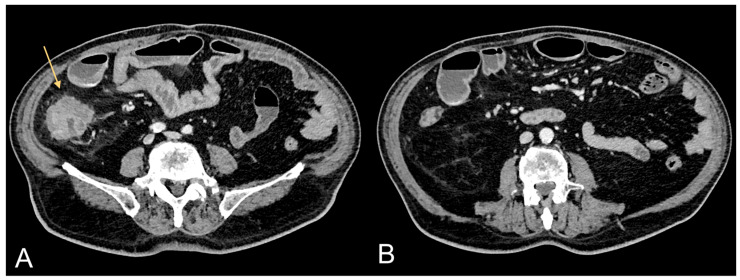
Contrast-enhanced CT images of a 75-year-old male patient showing CRC (light orange arrow in (A)) and associated excessive amount of VAT (B).
